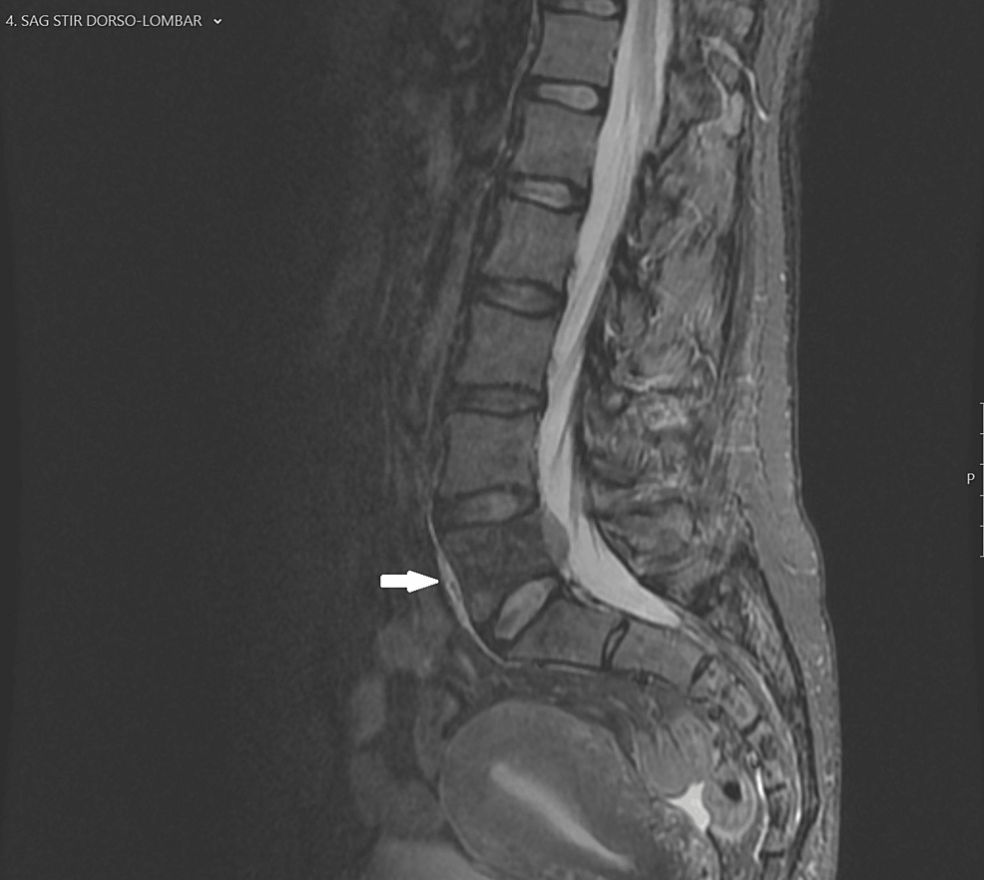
MRI, STIR sequence. On MRI, the lesion had no evident hypersignal on STIR sequences.
